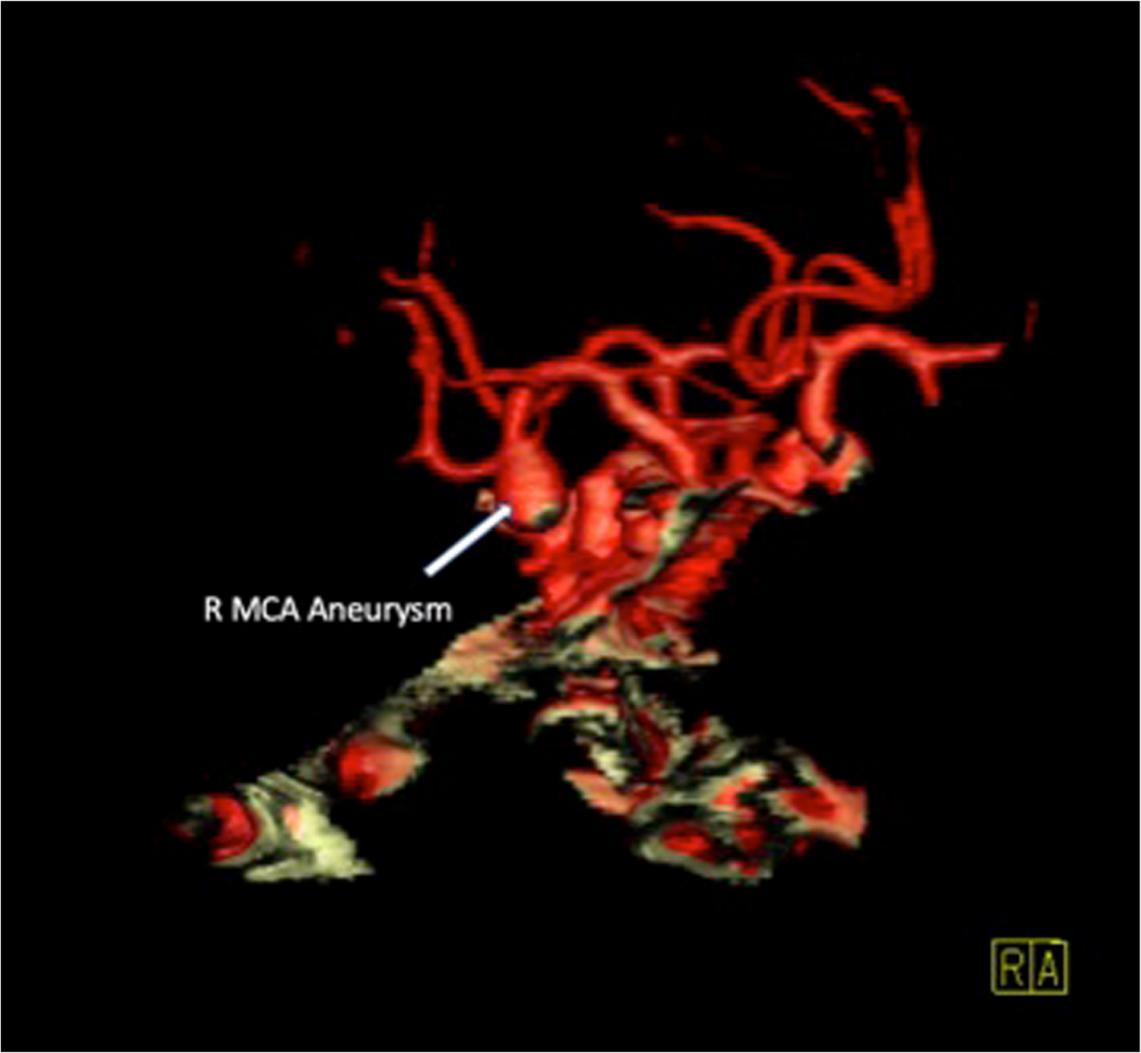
3D reconstruction of pre-operative CT angiogram. A 10 × 7 mm right MCA bifurcation aneurysm (white arrow) is shown
